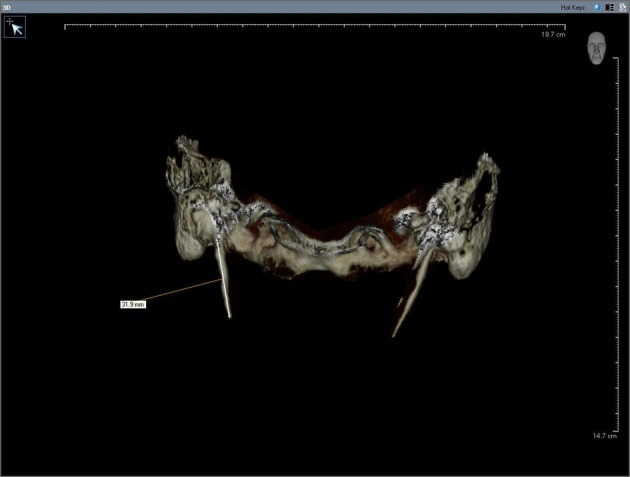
The length of the two segmented ossified SHC on CBCT
